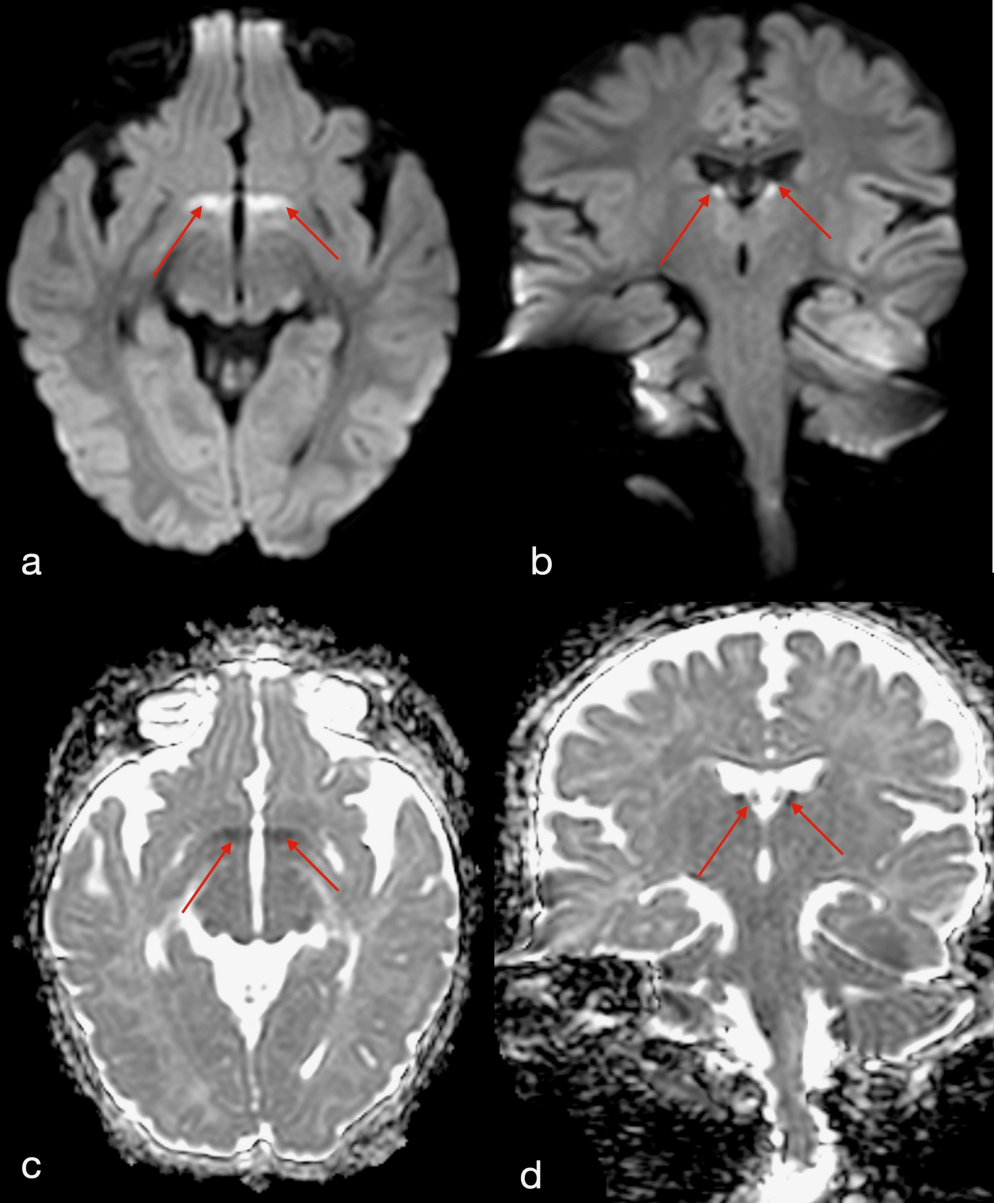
Axial and coronal DWI and ADC brain MRI of the anterior commissure Axial and coronal images demonstrate hyperintense signal on DWI (a and b) and loss of signal on ADC (c and d) in the bilateral anterior commissure (red arrows) to suggest restricted diffusion. DWI: diffusion-weighted imaging; ADC: apparent diffusion coefficient
